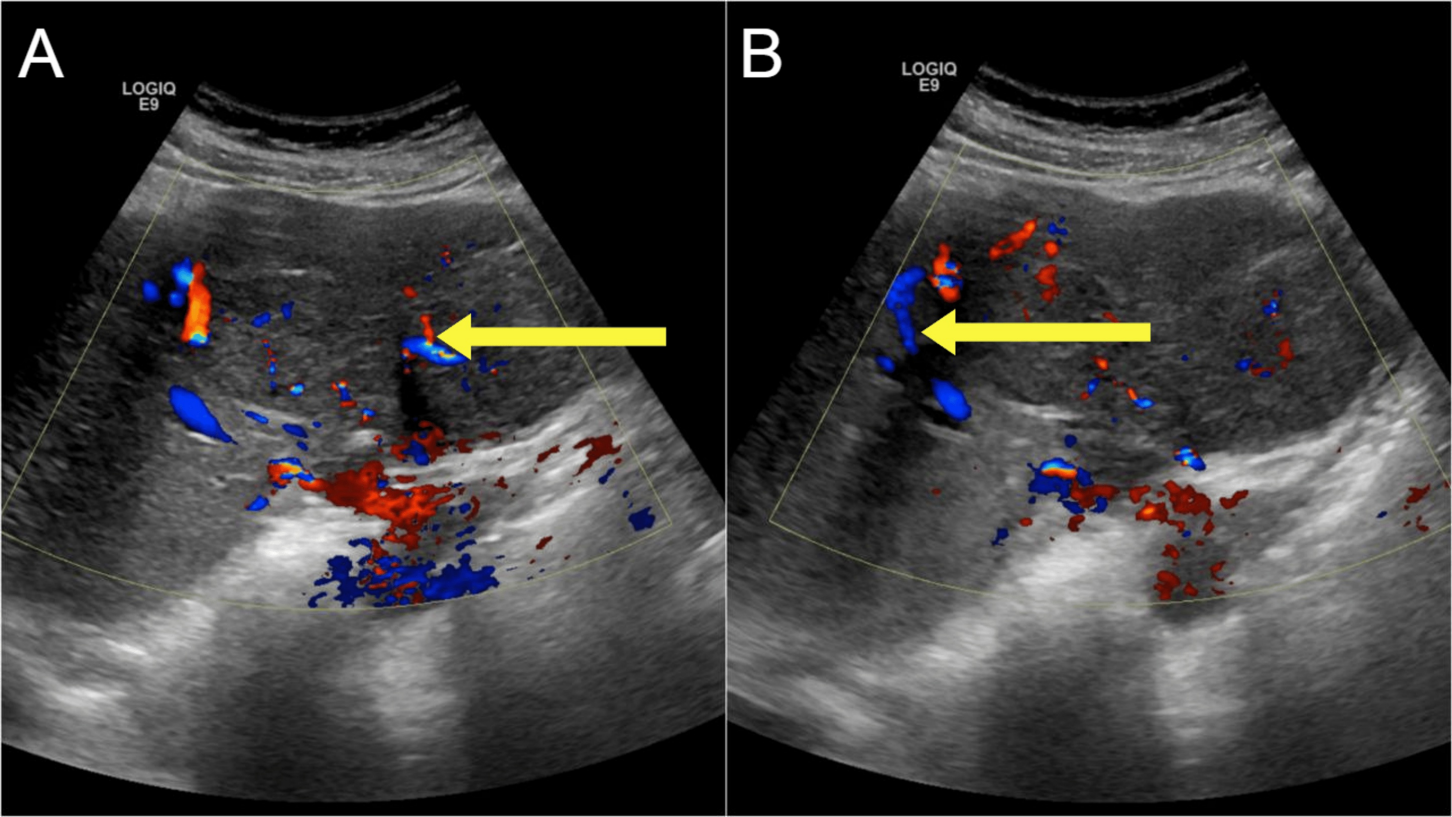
(A) Doppler ultrasound demonstrating the central vascular supply. (B) Doppler ultrasound demonstrating peripheral displacement of the vasculature.
